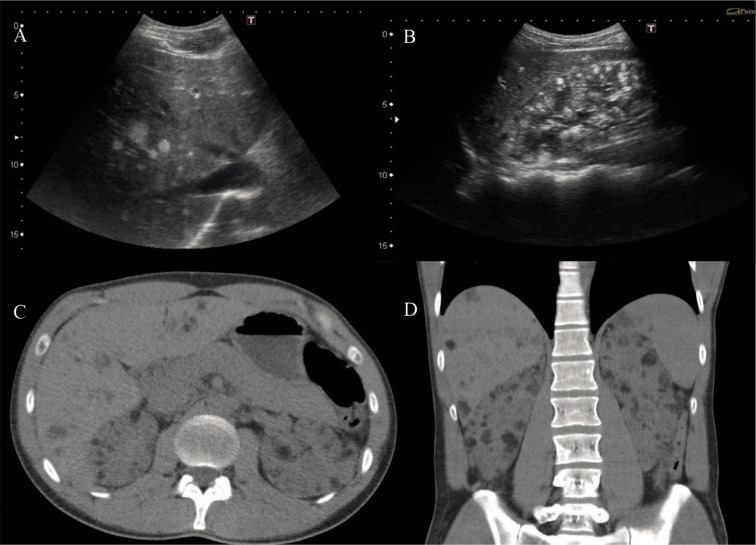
Images A and B: Ultrasonographic study showing multiple well-defined hyperechoic focal lesions diffusely distributed throughout the hepatic (A) and renal (B) parenchyma. Images C and D: Abdominopelvic CT without contrast, axial (C) and coronal (D) cuts, where multiple hypodense focal lesions with fat attenuation (< -20 UH) are visualized, diffusely distributed throughout the hepatic and renal parenchyma. Lesions are consistent with angiomyolipomas.
